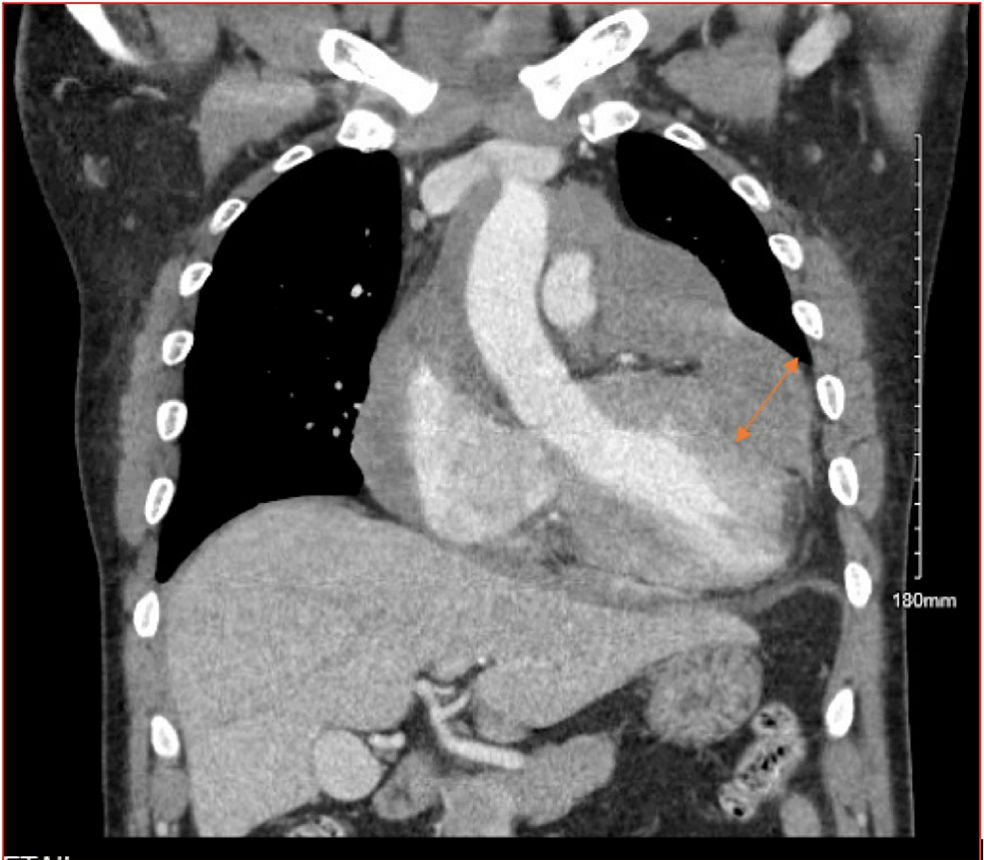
CT of the chest with contrast (coronal view) showing moderate-sized loculated pericardial effusion laterally that appears heterogenous.
